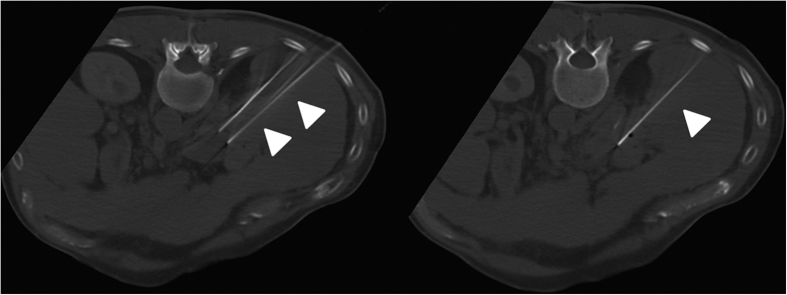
Same patient as in Figs 1 and 2 with HCC. Control CT during irreversible electroporation of HCC mass posterior to the main stem of the portal vein. The intervention required the patient to be placed in prone position in order to insert the 3 electrodes (tips of arrows).
